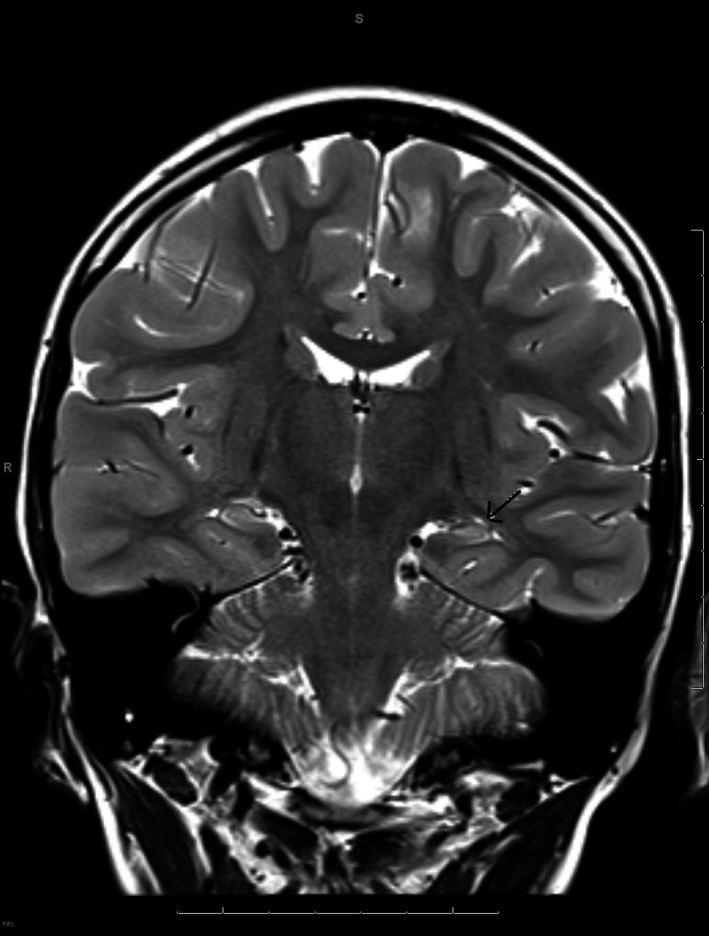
Epilepsy protocol MRI brain through hippocampi after 4 y: Coronal T2 sequence through hippocampi demonstrate a “layered” pattern of alternating T2 hyperintensity and isointensity involving the head and body of hippocampus(arrow)
